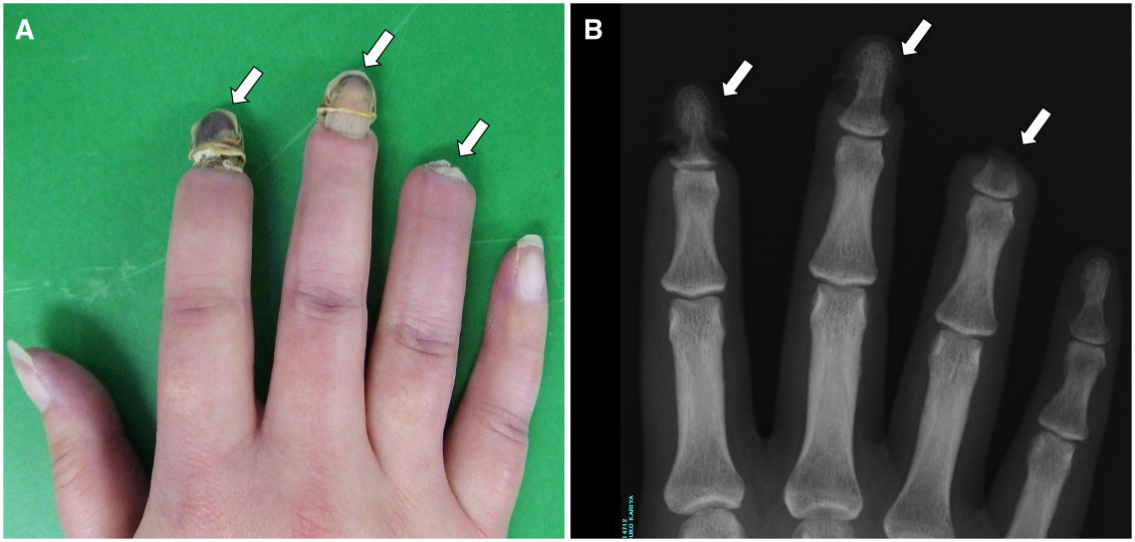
Physical and X-ray findings of systemic sclerosis associated acro-osteolysis.
